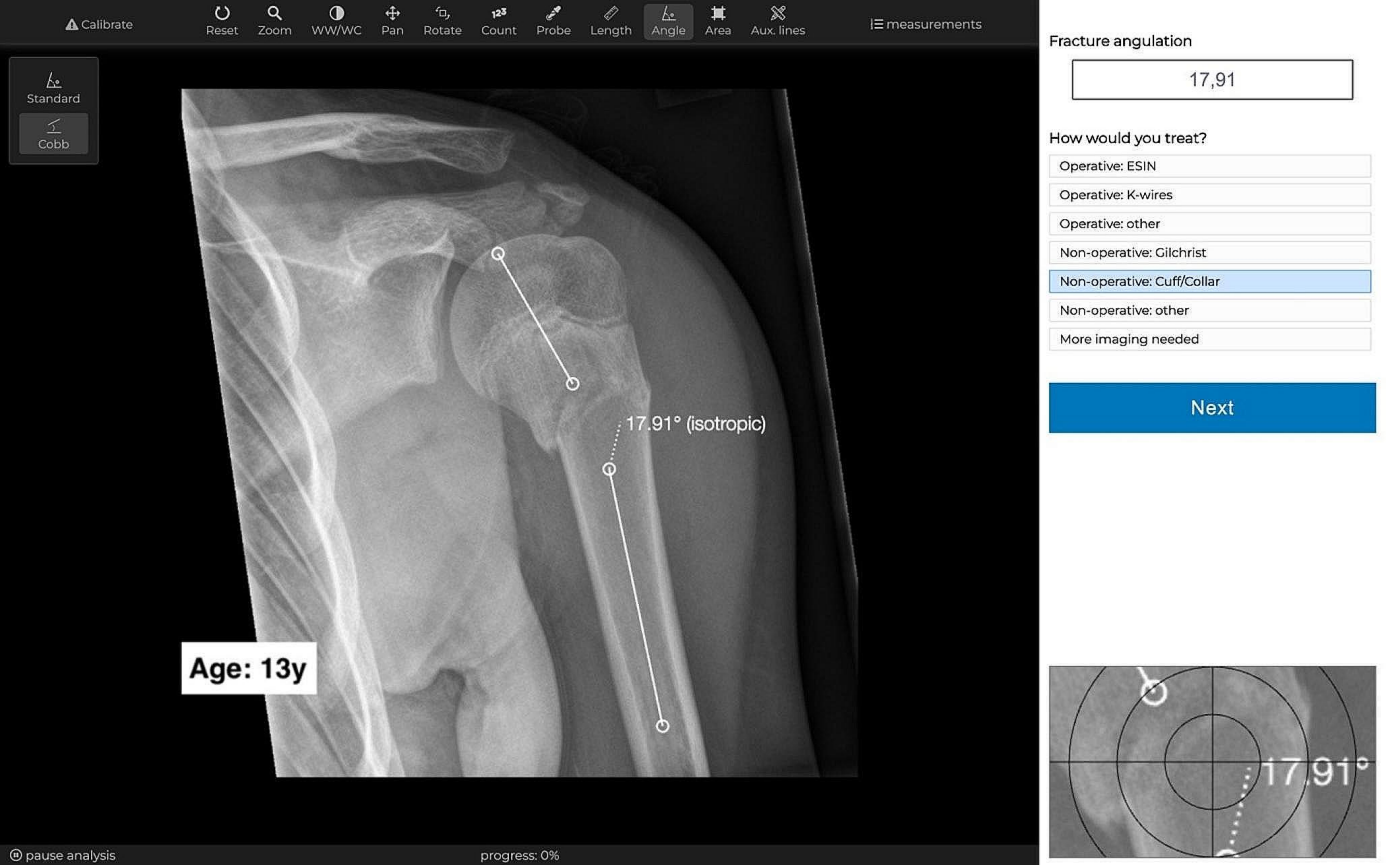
Image analysis was performed blinded and in random order using the online-tool Tyche Twenty-two experts in pediatric traumatology assessed twenty radiographs twice using the online-tool Tyche. Images were shown in random order with standard tools for analysis. On the same window was an input field to store the fracture angulation and a single-choice question with seven answers to choose operative and non-operative treatment. Results were immediately visible to the project manager
